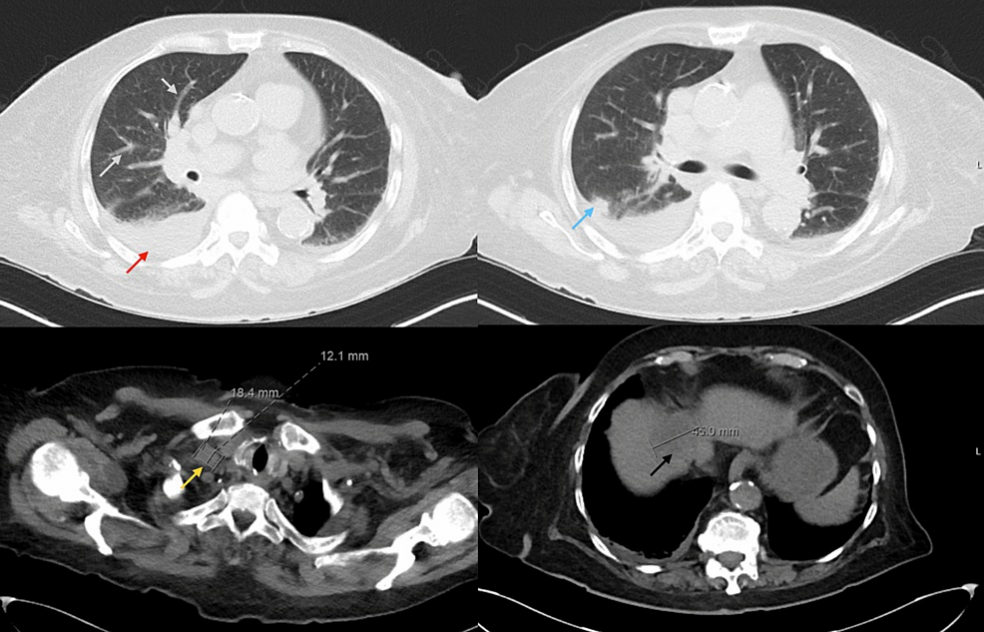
Computed tomography of the chest and abdomen without contrast Moderate right pleural effusion (red arrow), 1.4 cm ground glass nodule of the right upper lobe (blue arrow), bronchovascular thickening (grey arrow), right paratracheal lymph node (yellow arrow), and large hypodensity of the liver (black arrow).
